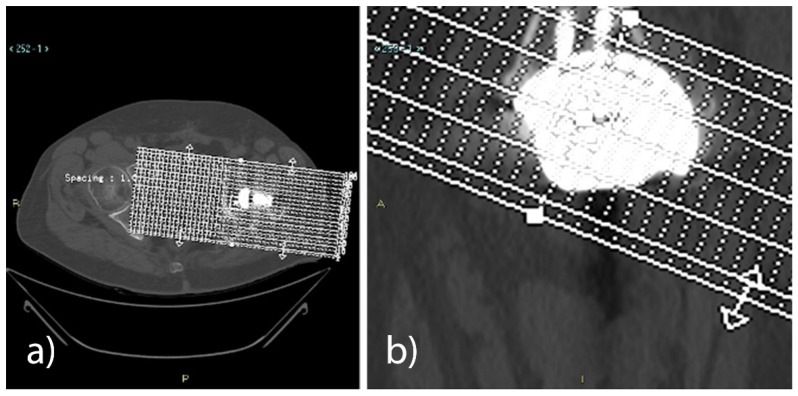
(a) Axial and (b) coronal CT views for bone gap measurement.
